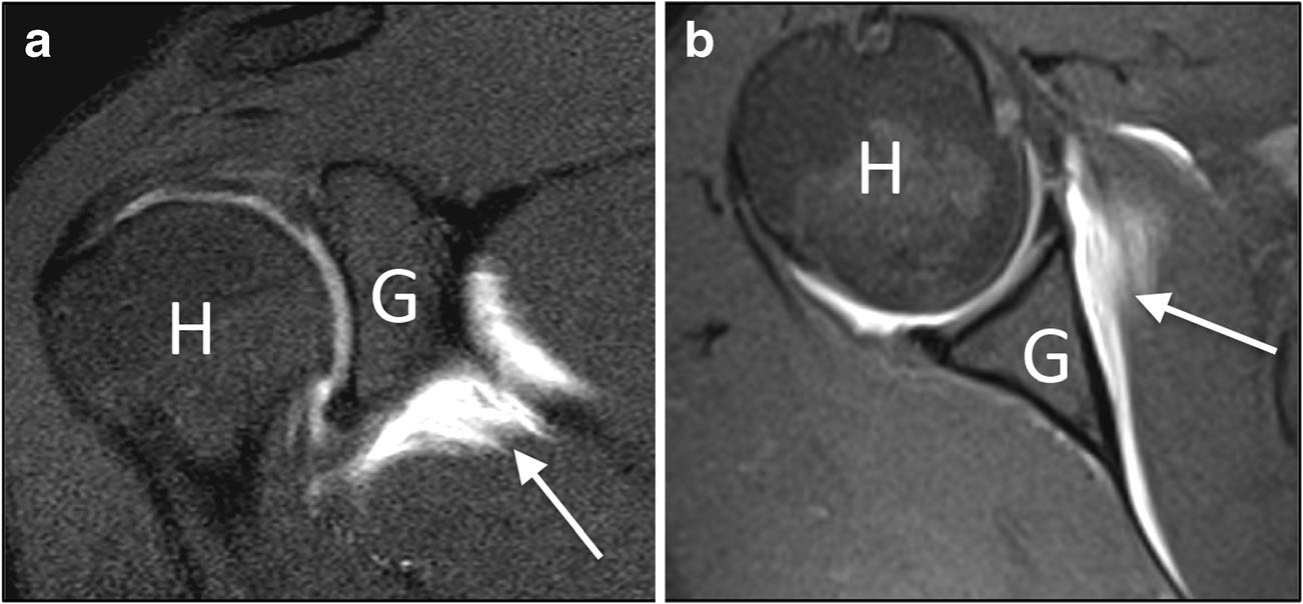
MR arthrography, (a) coronal oblique and (b) axial T1-weighted fat-saturated image in a patient with adhesive capsulitis. Leakage of contrast agent can be seen on the anterior inferior margin of the scapula (*arrow*). *H* humerus, *G* glenoid
